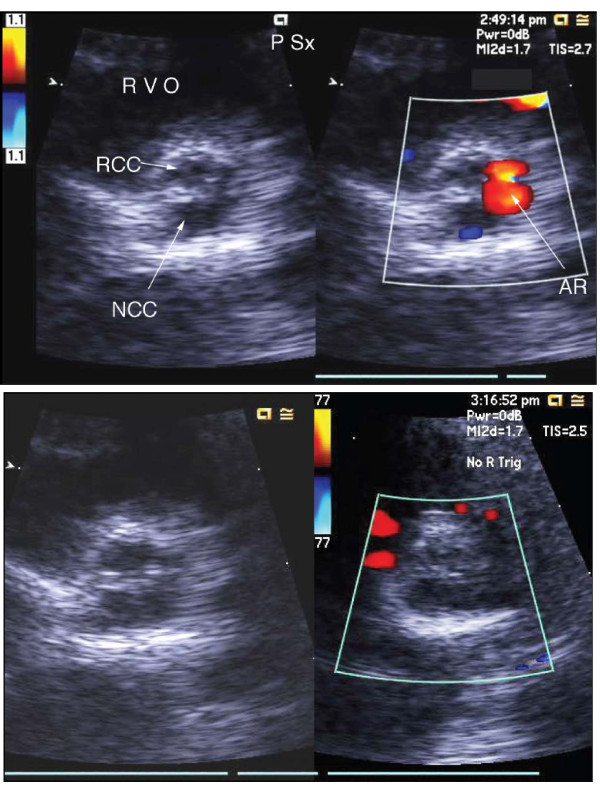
Severe aortic insufficiency seen on transthoracic echocardiography during catheterization. Severe aortic insufficiency is noted through the area of the left coronary cusp. Aortic insufficiency is terminated by placement of catheter into left coronary cusp.
